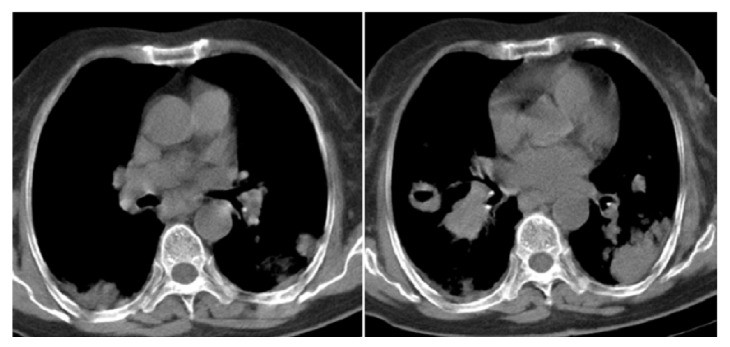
Mediastinal window of computed tomography scan showing bilateral mediastinal, hilar adenopathies, and cavitary lesions.
